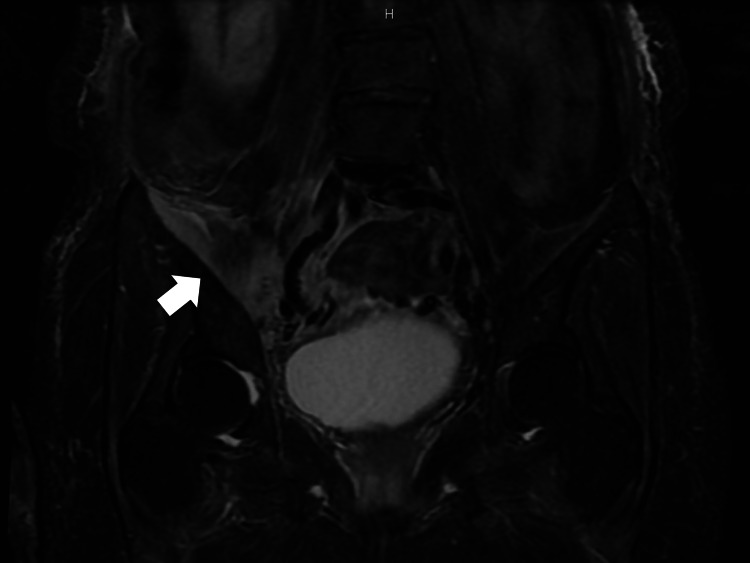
Magnetic resonance imaging (MRI, short-TI inversion recovery (STIR)) of the lumbar and pelvic: showing inflammation of the iliopsoas muscle (white arrow)
